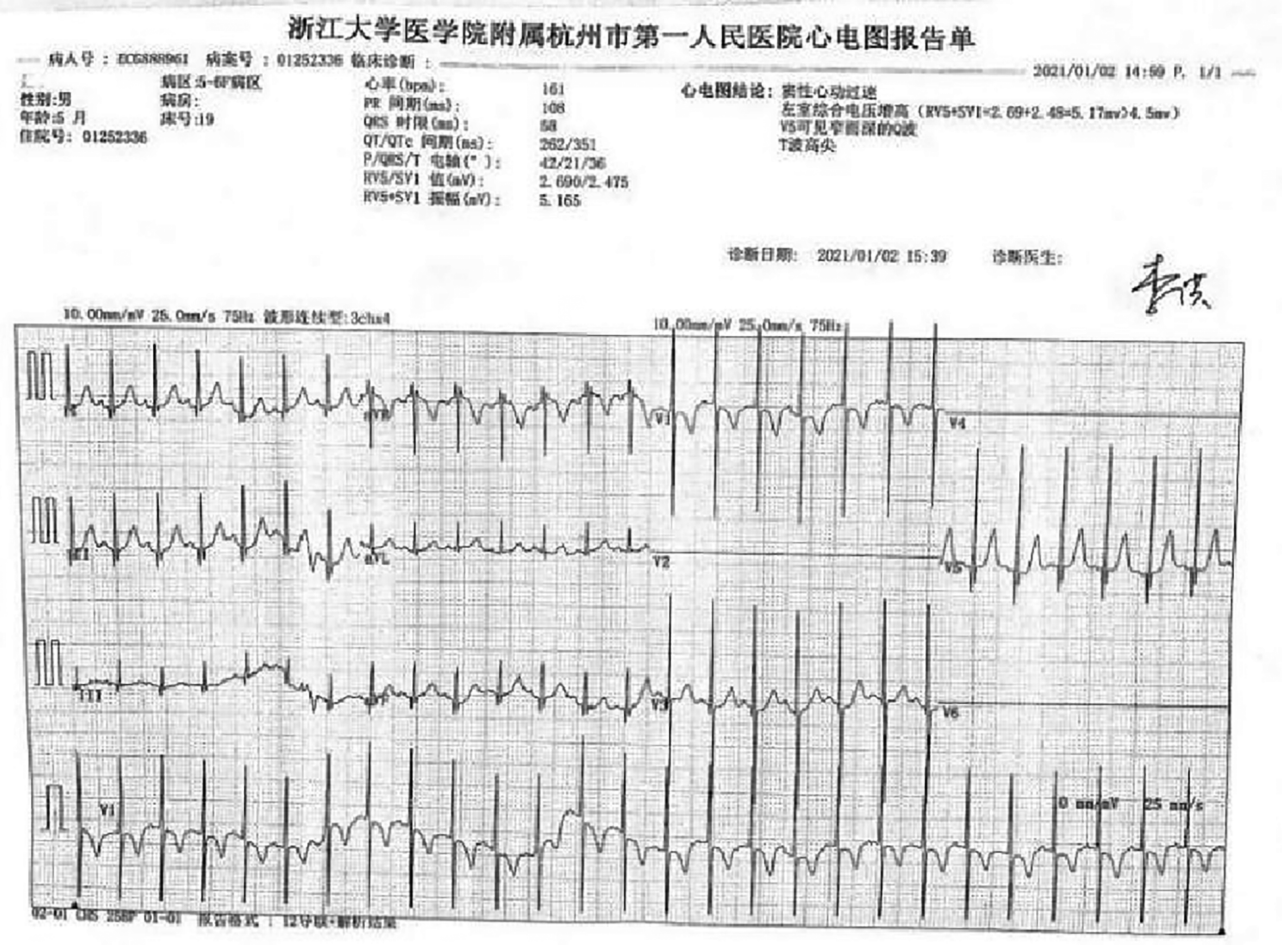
Echocardiography at 12 m.
